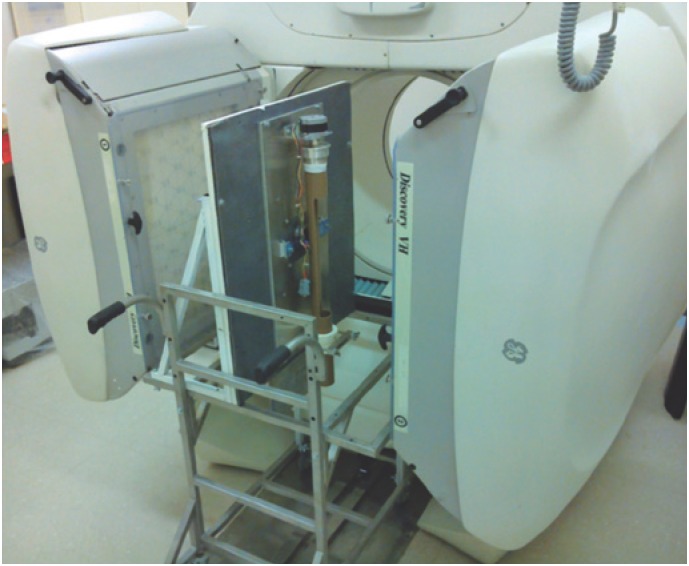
Image of the gamma-camara Discovery VH and the miniSPECT upgrading device
